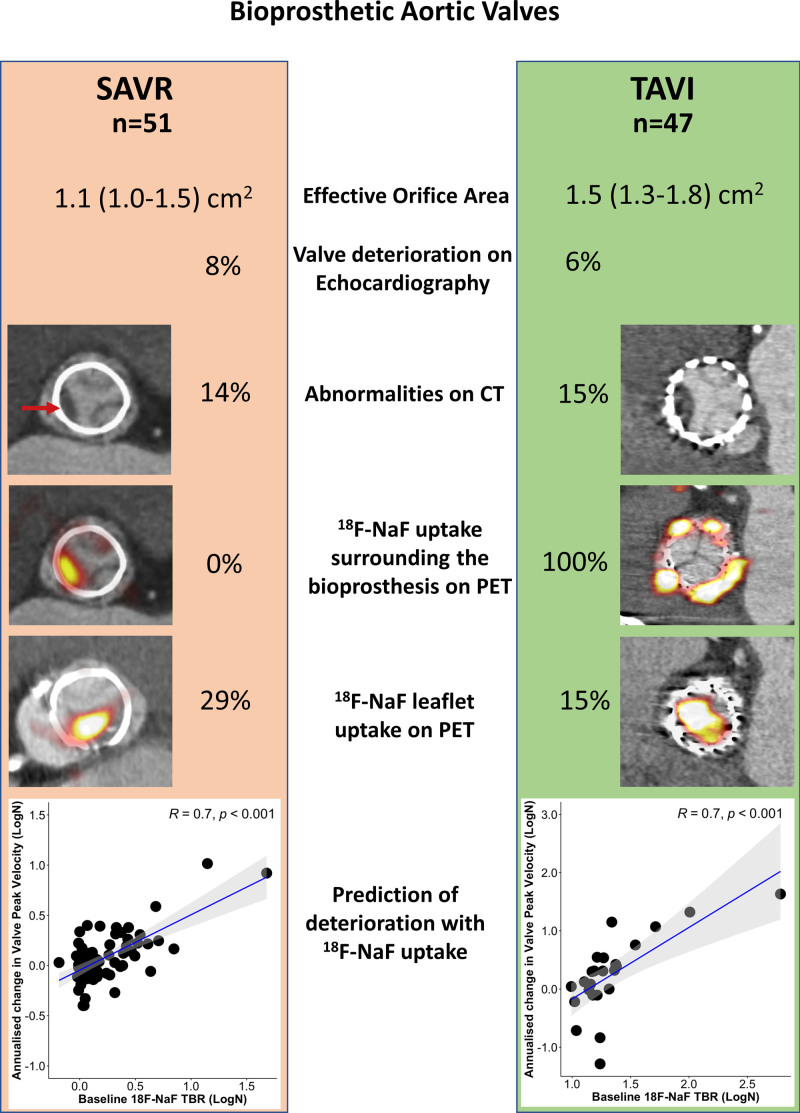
Comparison of imaging findings and valve deterioration in TAVI vs bioprosthetic SAVR. We compared echocardiographic, computed tomography (CT) and ^18^F-sodium fluoride (^18^F-NaF) findings in 47 patients with transcatheter aortic valve implantation (TAVI) with 51 patients with surgical aortic valve replacement (SAVR) who underwent the same research imaging protocol. We observed ^18^F-NaF uptake on the peripheral of all TAVI valves and none of the SAVR valves. Although patients with TAVI showed lower peak velocity (2.4 [2.0–2.7] vs 2.7 [2.4–3.0] m/s; *P*=0.03) and larger effective orifice area (1.5 [1.3–1.8] vs 1.1 [1.0–1.5] cm^2^; *P*=0.02) than patients with SAVR, we detected baseline echocardiographic (6% vs 8%; *P*=0.78) and CT abnormalities (15% vs 14%; *P*=0.87) suggestive of bioprosthetic degeneration in a similar proportion of patients with either TAVI or SAVR. The overall prevalence of patients with increased leaflet ^18^F-NaF uptake was nearly double in patients with SAVR compared with those with TAVI (29% and 15%; *P*=0.09). In both patients with SAVR or TAVI, baseline ^18^F-NaF leaflet uptake was predictive of the change in the peak transvalvular velocity on echocardiography. PET indicates positron emission tomography; and TBR, target-to-background ratio.
